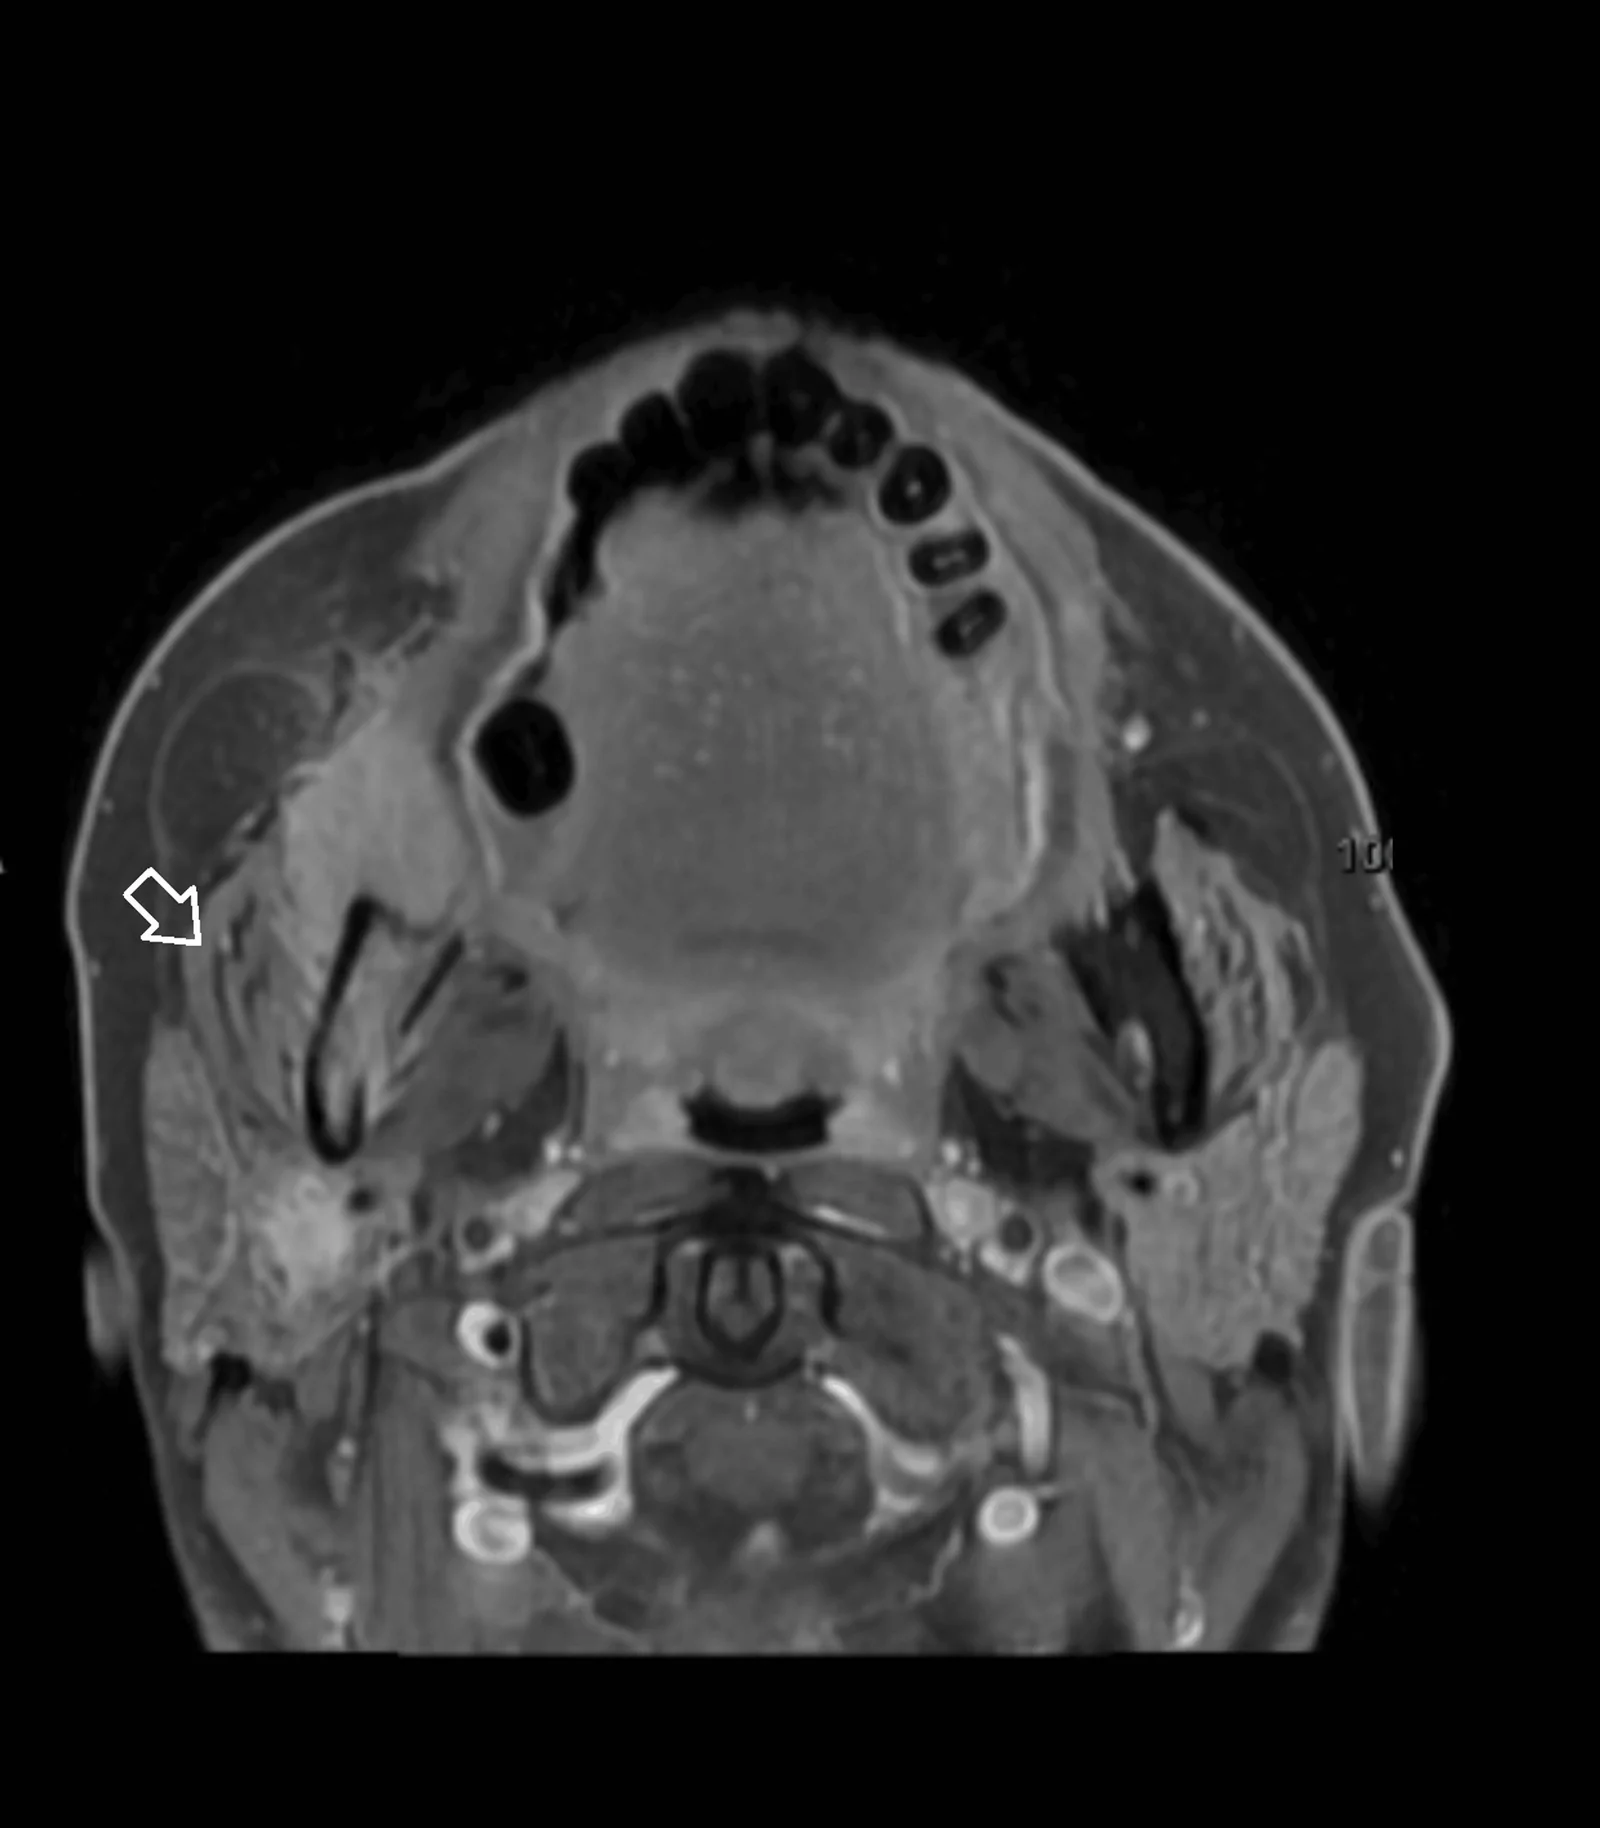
Coronal T2-weighted magnetic resonance imaging (MRI) demonstrating an infiltrative lesion centered in the right masticator space. The white arrow indicates the infiltrative soft tissue lesion extending toward the deep lobe of the right parotid gland.
